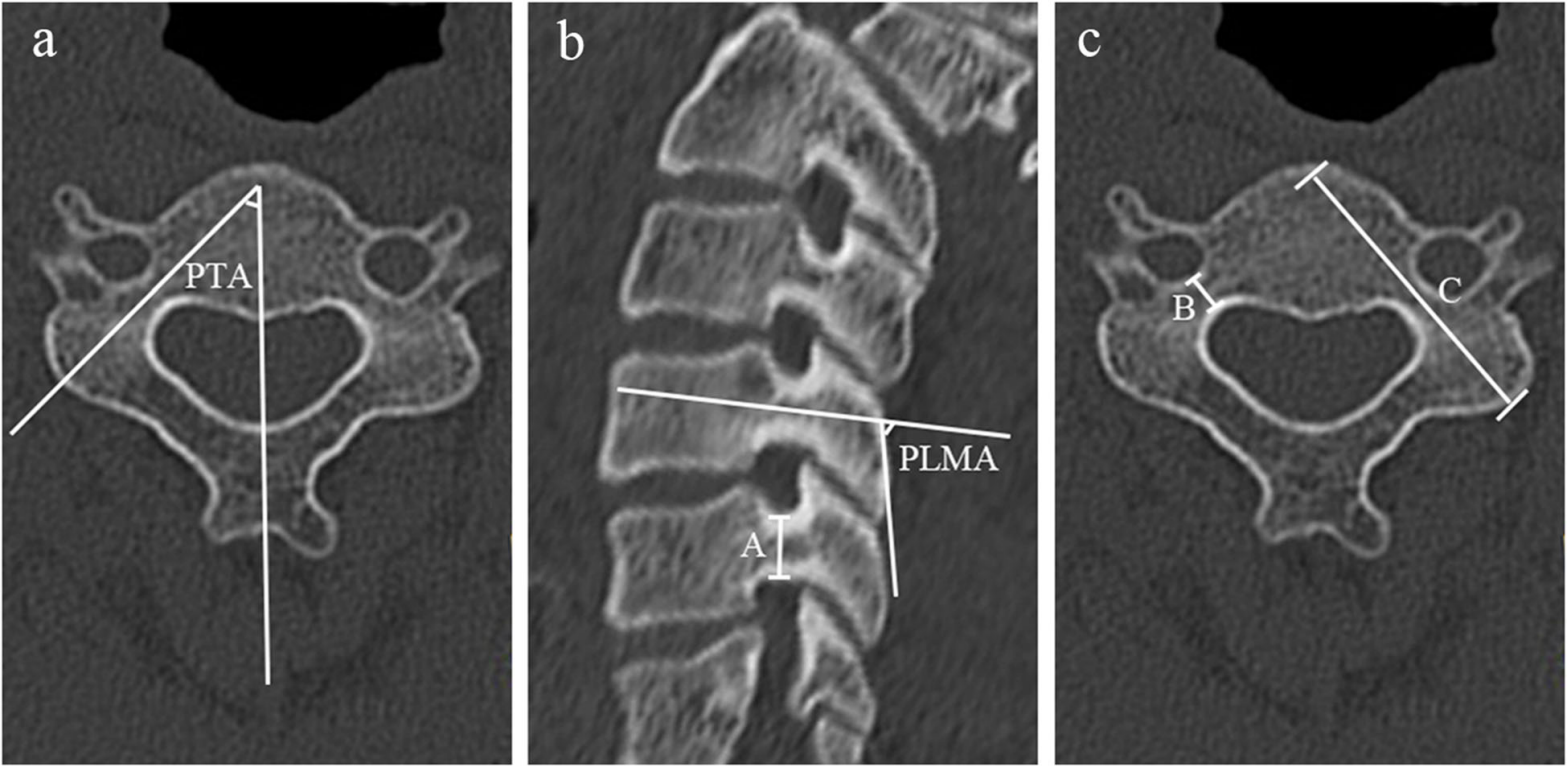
The preoperative pedicle transverse angle (PTA) and pedicle-lateral mass angle (PLMA) were measured on the transverse and sagittal CT images, respectively (a, b), and other parameters, including the pedicle height (A), pedicle width (B) and pedicle axis length (C), were also measured (b, c). The PTA referred to the included angle between the pedicle trajectory and the vertical line of the posterior edge of the vertebral body on the transverse CT images, and the PLMA was defined as the included angle between the pedicle trajectory and the posterior edge of lateral mass on the sagittal CT images
